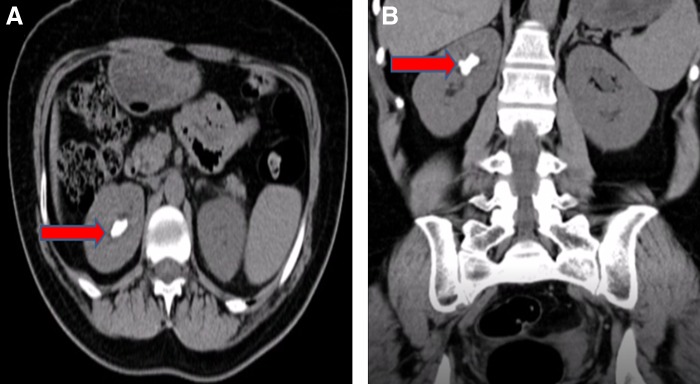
CT scan showing a 2.6 cm staghorn stone with 826 HU density in the right kidney (*red arrow*). (A) Axial view. (B) Coronal view.
